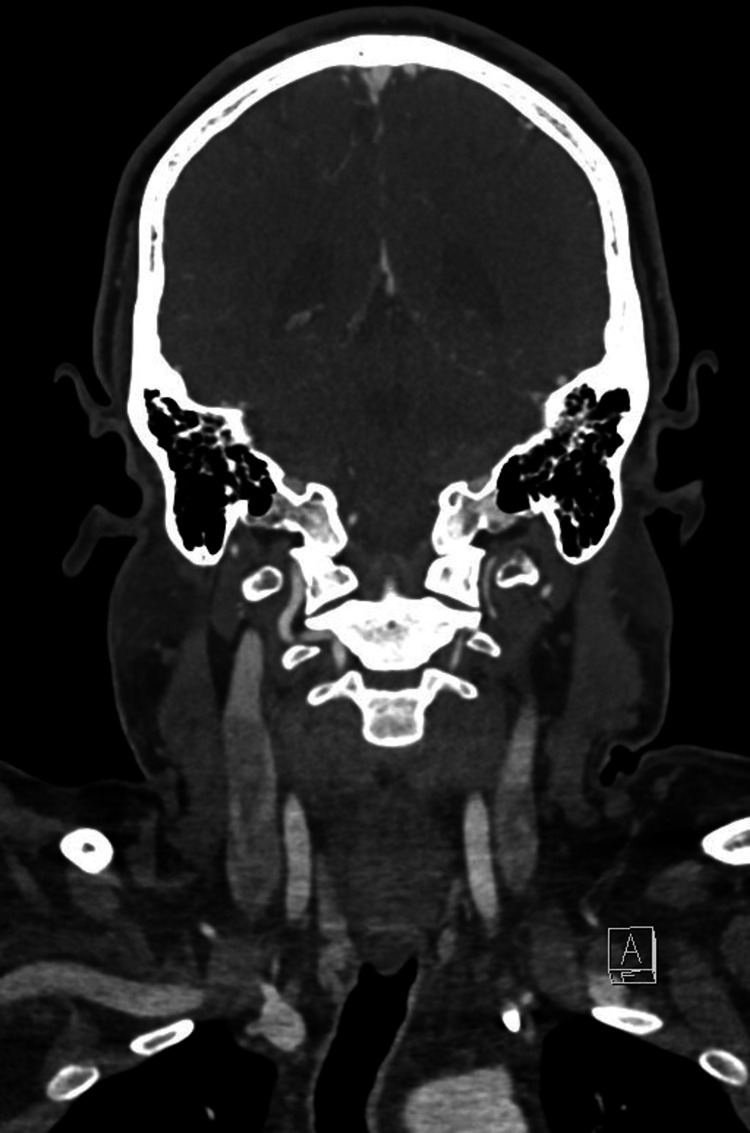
CTA of the head and neck demonstrating patent intracranial arterial vasculature, patent neck arterial vasculature, and no evidence of high-grade stenosis or dissection CTA: computed tomography angiography
